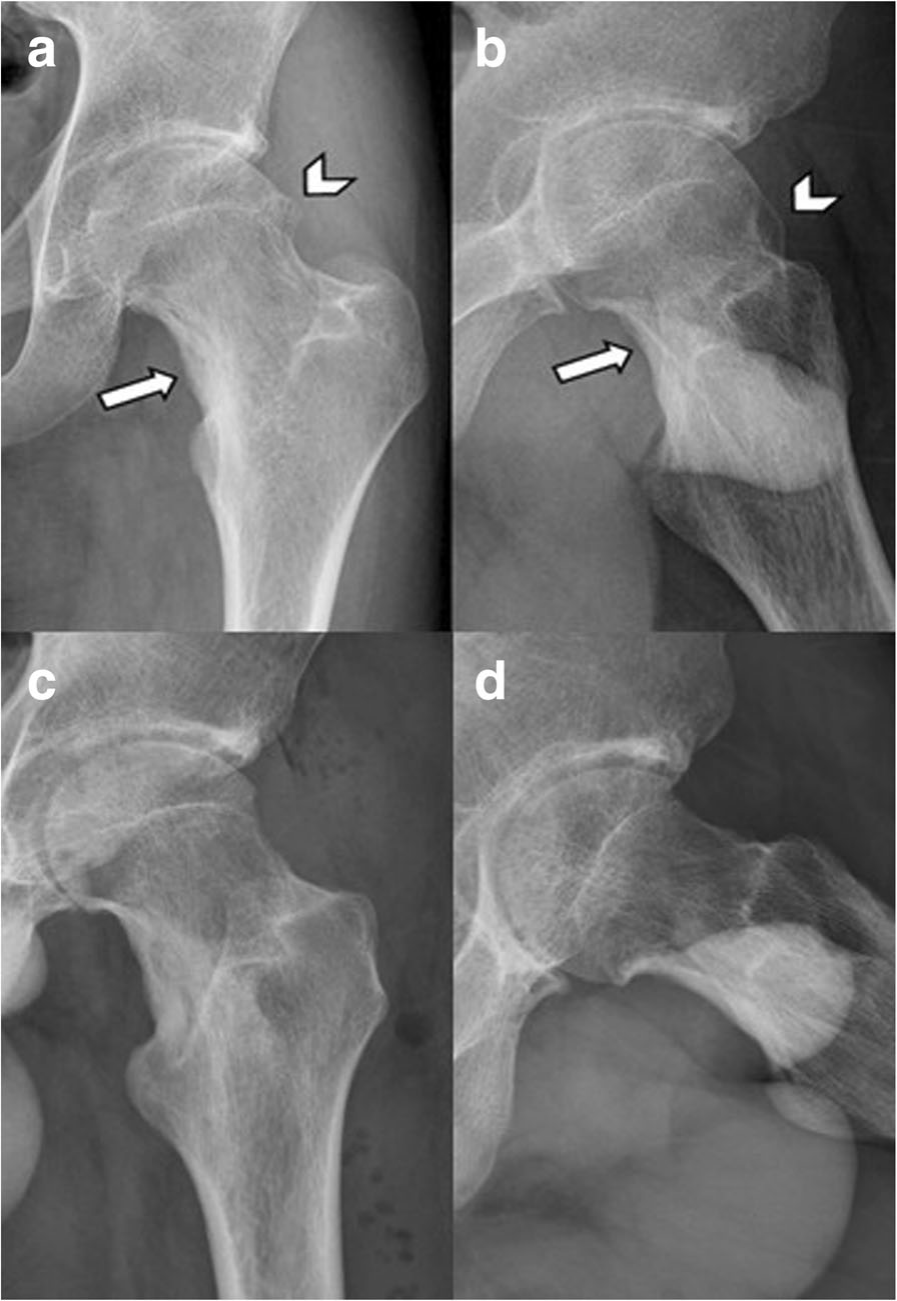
An 18-year-old male patient, who has undergone curettage and bone grafting for left femoral neck osteoid osteoma 2 years ago, presented with left proximal femur cam-type FAI. a, b Preoperative hip AP and lateral view showing the location of osteoid osteoma (arrow) and the bony bump (arrowhead). c, d Follow-up radiography at postoperative 2 years after complete removal of the cam lesion
